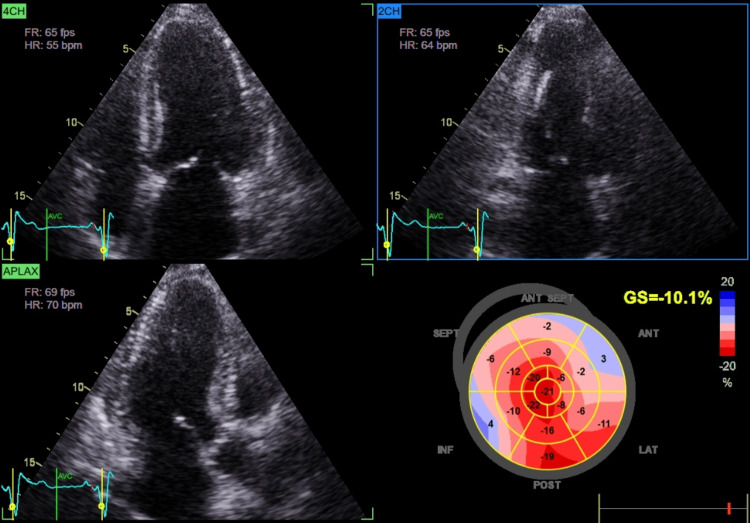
Transthoracic echocardiography demonstrating reduced global longitudinal strain (-10%) consistent with preserved EF (60%) EF: Ejection Fraction
